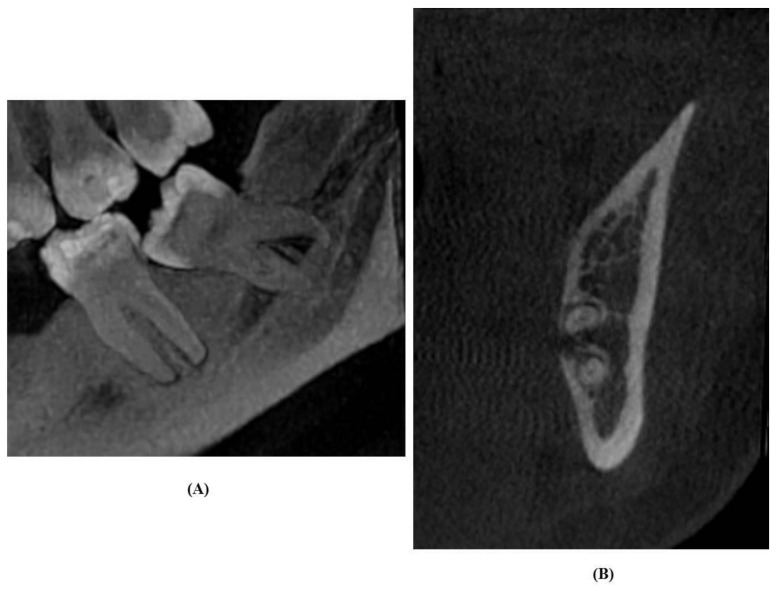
Panoramic images show darkening of the roots (A) and lingually positioned inferior alveolar canal with cortication on CBCT images (B).
